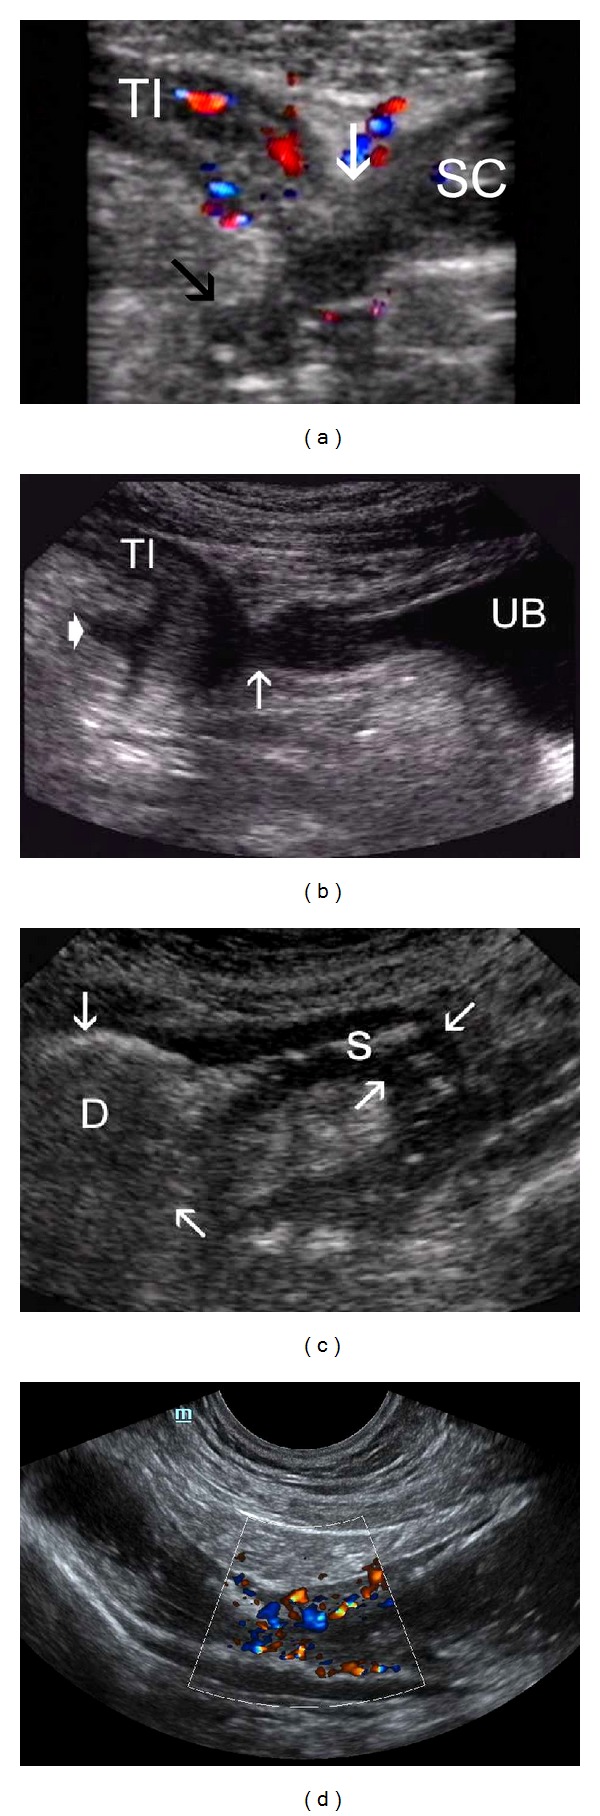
Crohn's disease complications. (a) Transverse view in lower abdomen shows fistula (white arrow) between terminal ileum/TI) and sigmoid colon (SC), black arrow points to small abscess, *high resolution probe*. (b) Oblique section of terminal ileum (TI) with blind fistula (thick arrow) into echogenic mesenterial fat and ileovesical fistula (thin arrow). *Standard abdominal probe*. (c) Stricture of ileum (S) with prestenotic dilatation (D)—*standard abdominal probe*. (d) TUS with color doppler and peroral contrast—Crohn's terminal ileum stenosis with intramural hypervascularisation (with CFD) indicates inflammatory stenosis—*high resolution probe. *
